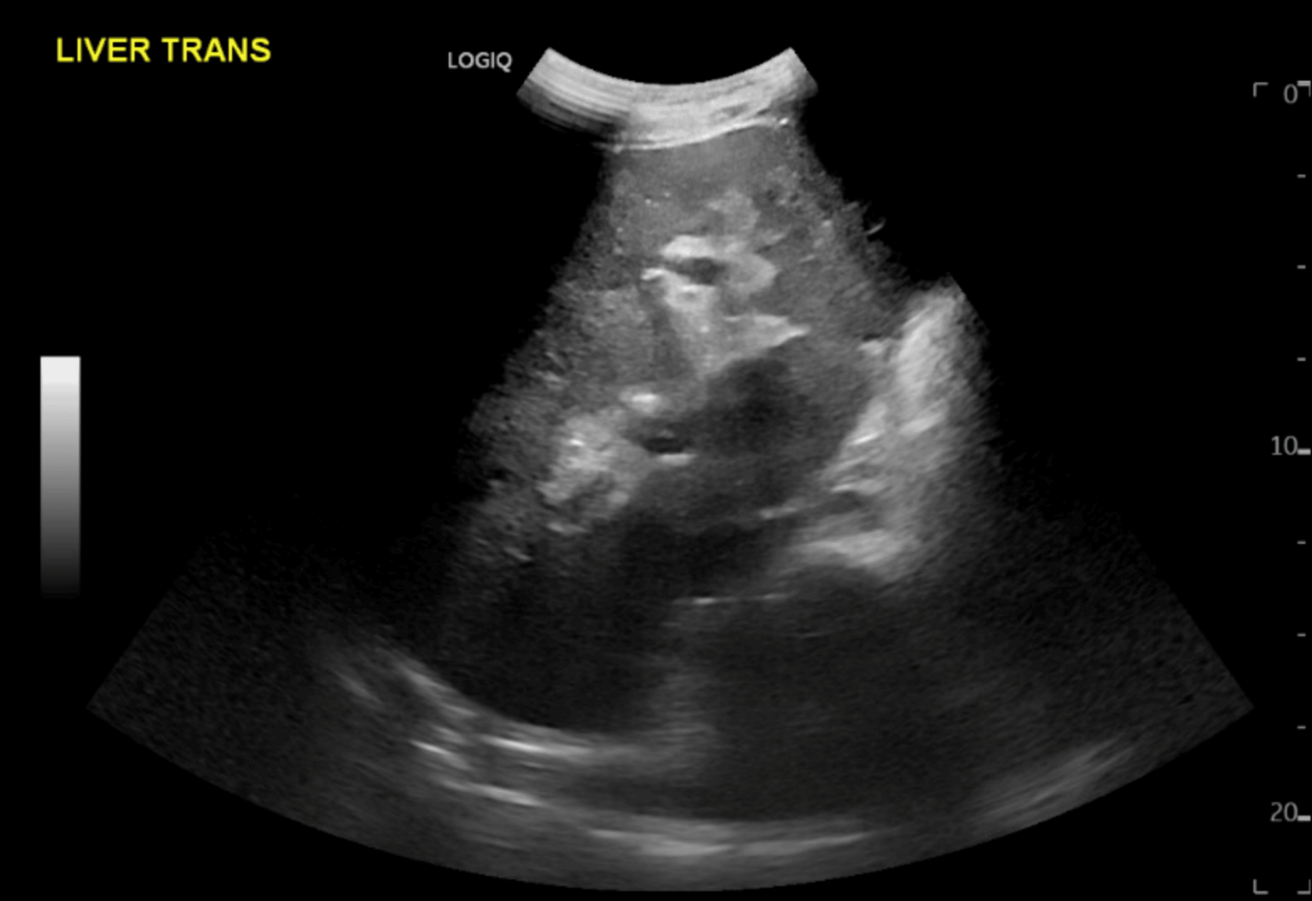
Altered hepatic architecture as seen on the repeat abdominal ultrasonography
